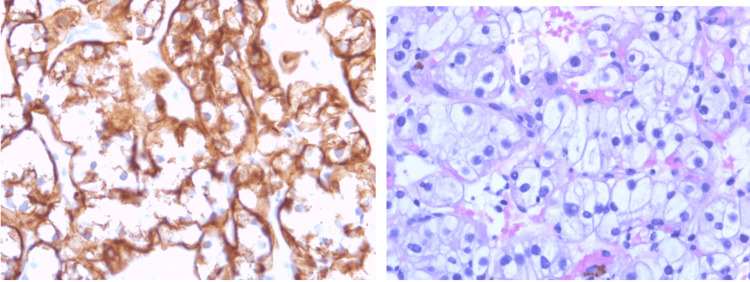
Pathology from ultrasound-guided left renal biopsy.
